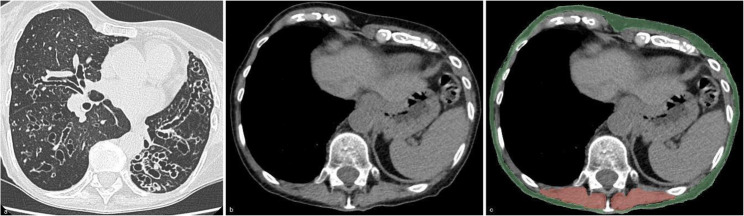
Axial chest CT of a 59-year-old female patient affected by severe bilateral bronchiectasis A. In (B), the soft tissue window at the 12th thoracic vertebra level which has been used for the segmentation. In (C), the segmentation of the subcutaneous tissue (green area) and paravertebral muscle (red area)
